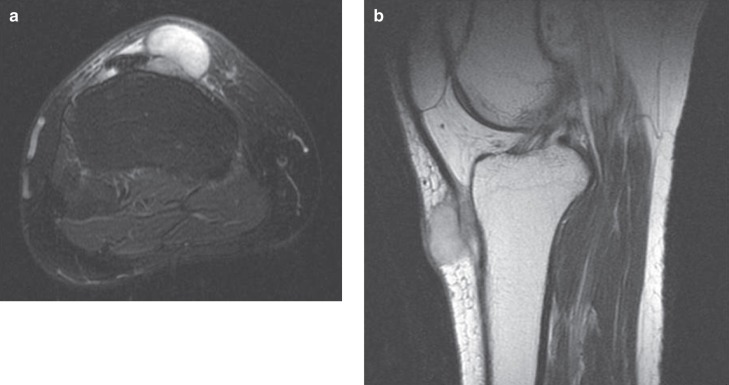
MRI after preoperative radiation therapy. The size of the tumor was reduced, and the high signal intensity area was decreased.
